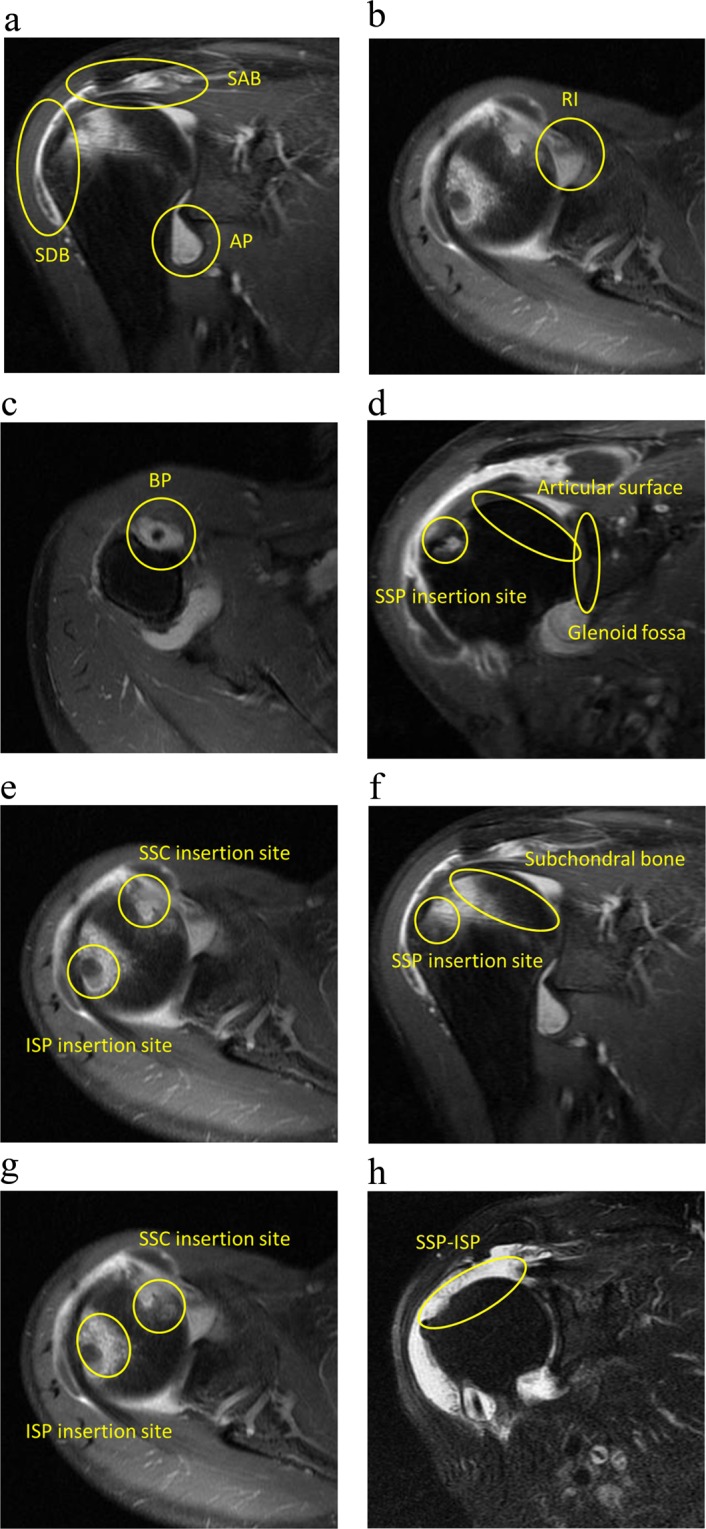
The inflammatory changes on MRI. The shoulder images are Gd-DTPA T1 FS sequences showing the synovitis (a–c), the erosion (d,e), the bone edema (f,g), and oblique coronal T2 FS showing the rotator cuff tear (h). Gd-DTPA: gadolinium-diethylenetriamine pentaacetic acid, T1 FS: T1-weighted fast spin-echo sequences with frequency-selective fat saturation, T2 FS: T2-weighted fast spin-echo sequences with frequency-selective fat saturation, SAB: sub-acromial bursa, SDB: sub-deltoid bursa, AP: axillary pouch, RI: rotator interval, BP: biceps pulley, SSP: supraspinatus, ISP: infraspinatus, SSC: subscapularis.
